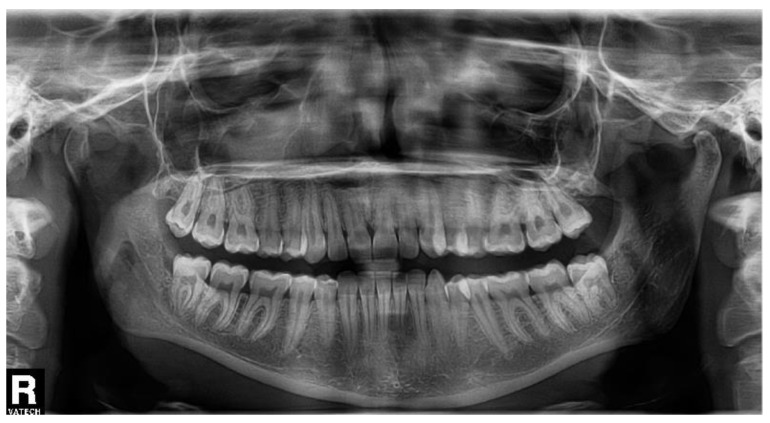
The orthopantomogram of the patient 3. Tooth No. 38 is in normal position, with a semilunar burnout distally.
